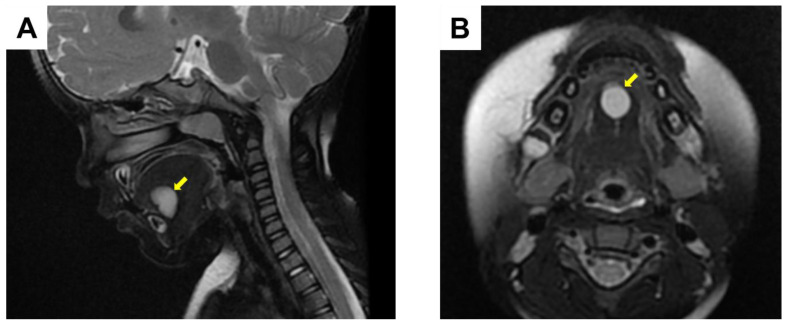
MRI results at time of initial visit. Arrow indicates cystic lesion. (A) Axial T2-weighted image. (B) Coronal T2-weighted image.
